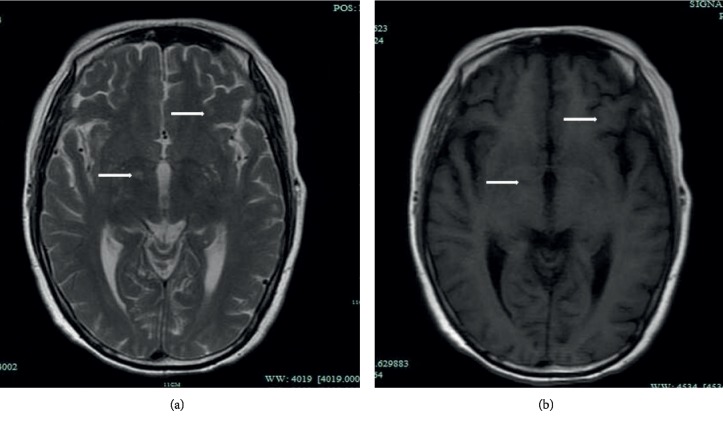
A 53-year-old woman with cryptococcal meningitis with a history of KT 12 years ago. Brain MRI shows local lesions in the right basal ganglion and frontal lobe. (a) Axial T2-weighted image showing a high signal. (b) Axial T1-weighted image showing a low signal.
